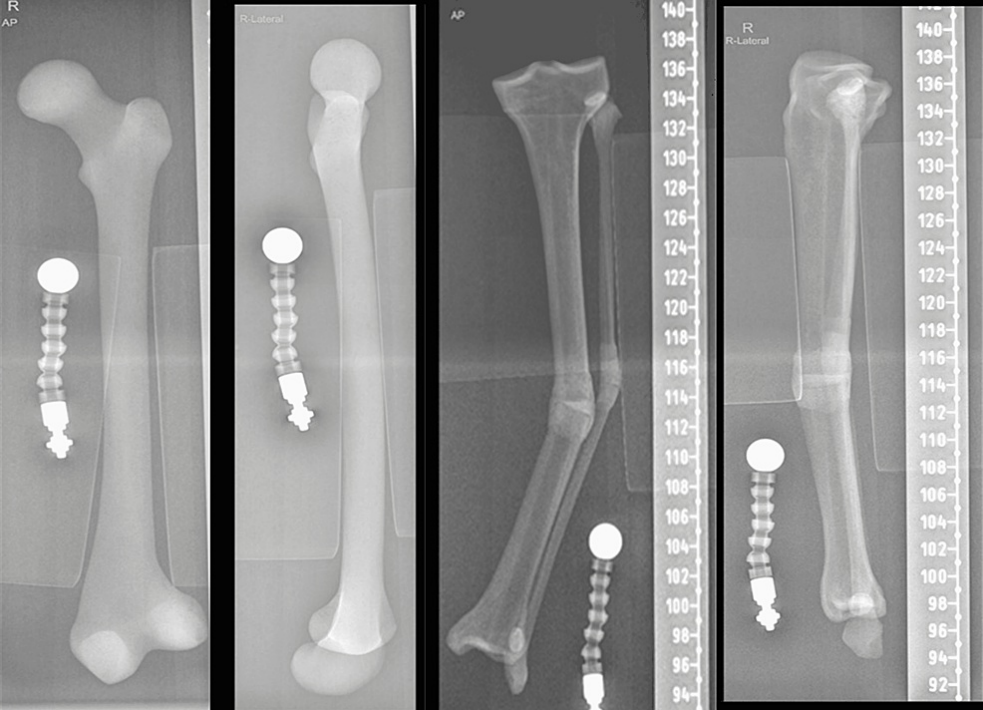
Plain radiographs of the Sawbones in Figure 2 using calibrating balls
